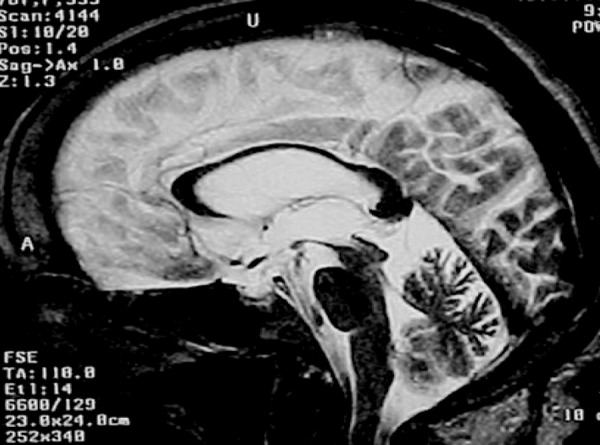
Sagittal T2-weighted images shows marked atrophy of the superior
